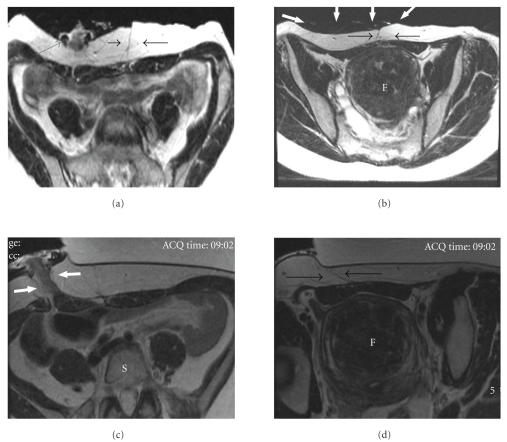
Pretreatment screening axial T2 weighted screening MR images (a, b) and axial T2 weighted images on the day of treatment (c, d). On screening images, the ostomy is evident in the right lower quadrant (grey arrows), and longitudinal abdominal scarring is seen in the subcutaneous tissue in the midline (black arrows) which overlies the uterine fibroid (F). The ostomy bag can also be seen to overlie the anterior abdominal wall (white thick arrows). On the day of treatment, the ostomy bag was placed off to the side and was no longer visible on the images. The skin of the anterior abdominal wall was pulled off to the right side such that the ostomy and scar are now out of the treatment window and the fibroid (F) is accessible for treatment.
